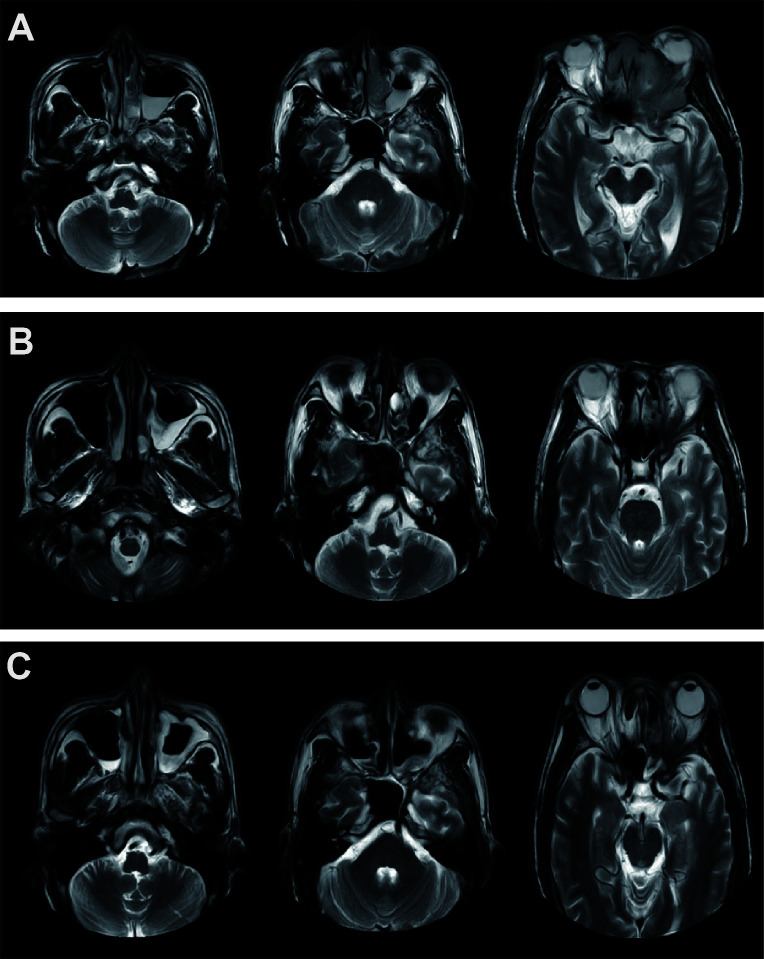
Imaging examinations of the patient during treatment. (A) MRI of the primary tumor before treatment. (B) MRI after three cycles of neoadjuvant therapy with nivolumab. (C) Imaging results following neoadjuvant therapy, surgery, and concurrent chemoradiotherapy.
